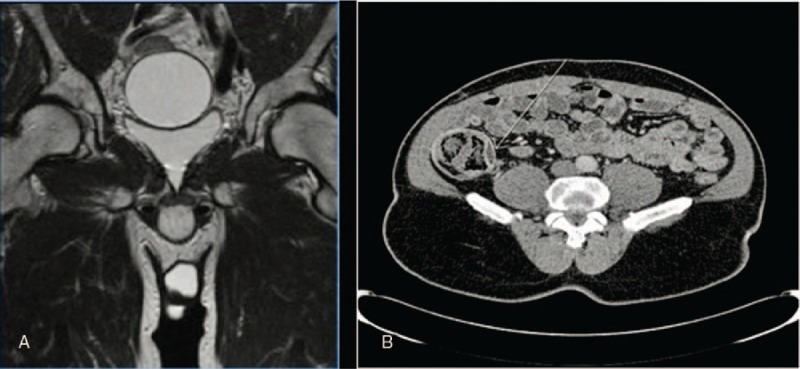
MRI showed the reservoir to be full and centrally placed within the pelvis (A). Abdominal CT scan revealed that the reservoir had migrated into the abdomen and had become wrapped around the caecum (B). CT = computed tomography, MRI = magnetic resonance imaging.
